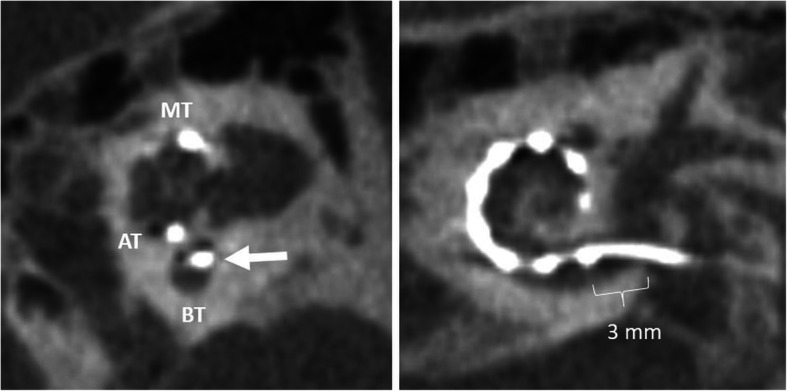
A 15-year-old male patient, with normal postoperative finding after CI surgery. CBCT paracoronal image (left image) shows an electrode array located in the scala tympani (lower segment) of the cochlear duct (white arrow). BT – basal turn. MT – middle turn. AT – apical turn. CBCT paraaxial image (right image) shows the most basal electrode contact for this type of implant correctly located 3 mm below the round window
